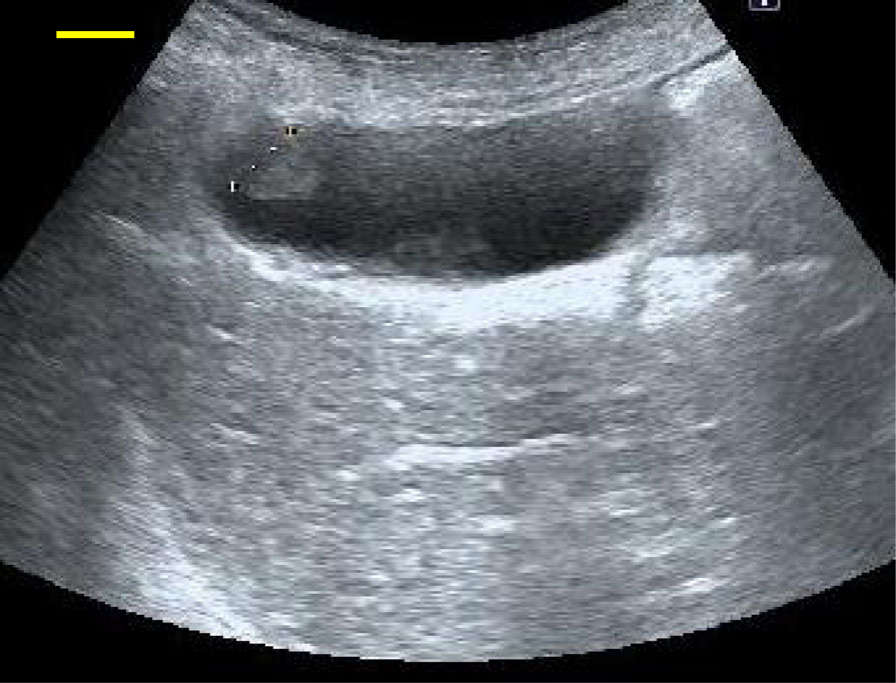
Ultrasonography shows an iso- to hyperechoic polyp at the fundus of the gallbladder (*yellow bar* indicates 10 mm)
